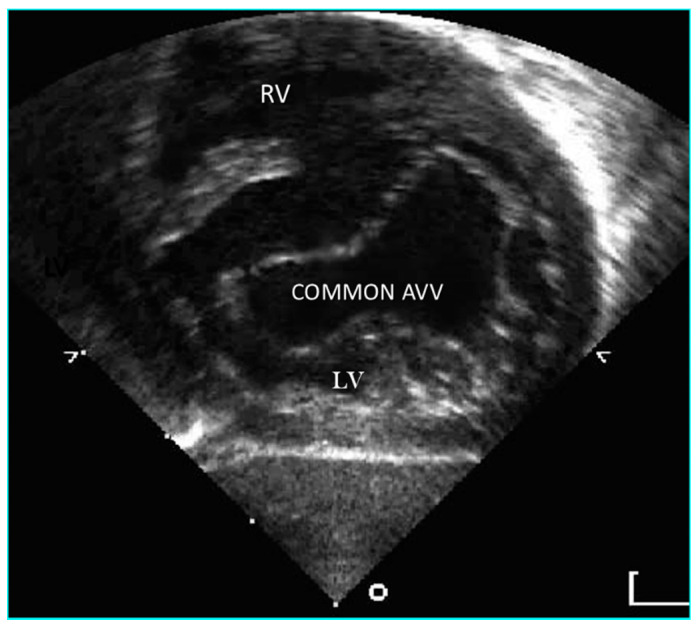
Subcostal left oblique echocardiographic section at the level of the atrioventricular junction showing the common atrioventricular valve committed entirely to the left ventricle.
